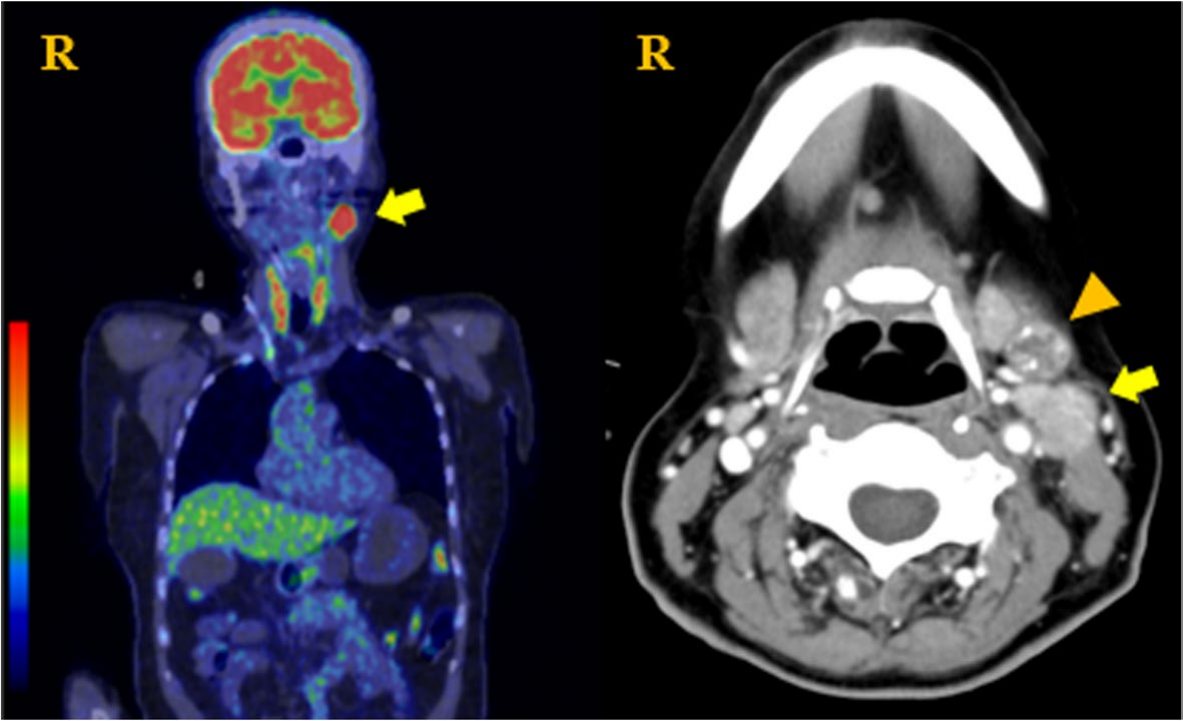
FDG-PET (fluorodeoxyglucose-positron emission tomography) and computed tomography (CT) images with contrast enhancement. FDG-PET showed abnormal hot spots in the left neck (arrow). CT images with contrast enhancement showed a tumor associated with the left submaxillary gland, showing contrast effects along the periphery (arrow) and an increase in lymph nodes with calcification (arrowhead)
